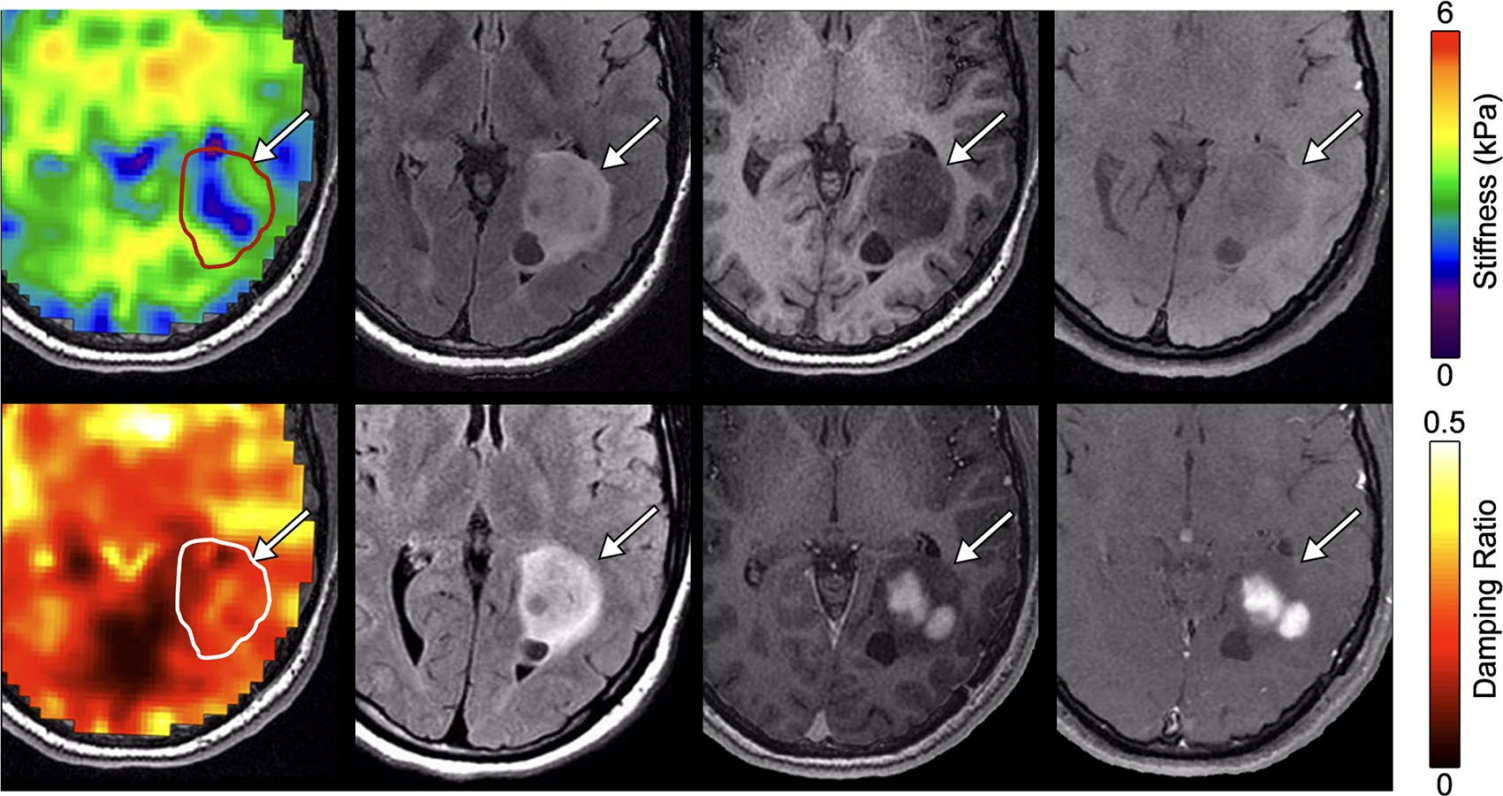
Large low-grade gliomas show mechanical heterogeneity. 16-year-old Female Pilocytic astrocytoma (Patient 1). Top row, left-to-right: Stiffness map, T_2_-CUBE, T_1_-BRAVO, MEMP; Bottom row, left-to-right: Damping ratio map, T_2_-FLAIR, post contrast T_1_-BRAVO, post contrast MEMP
